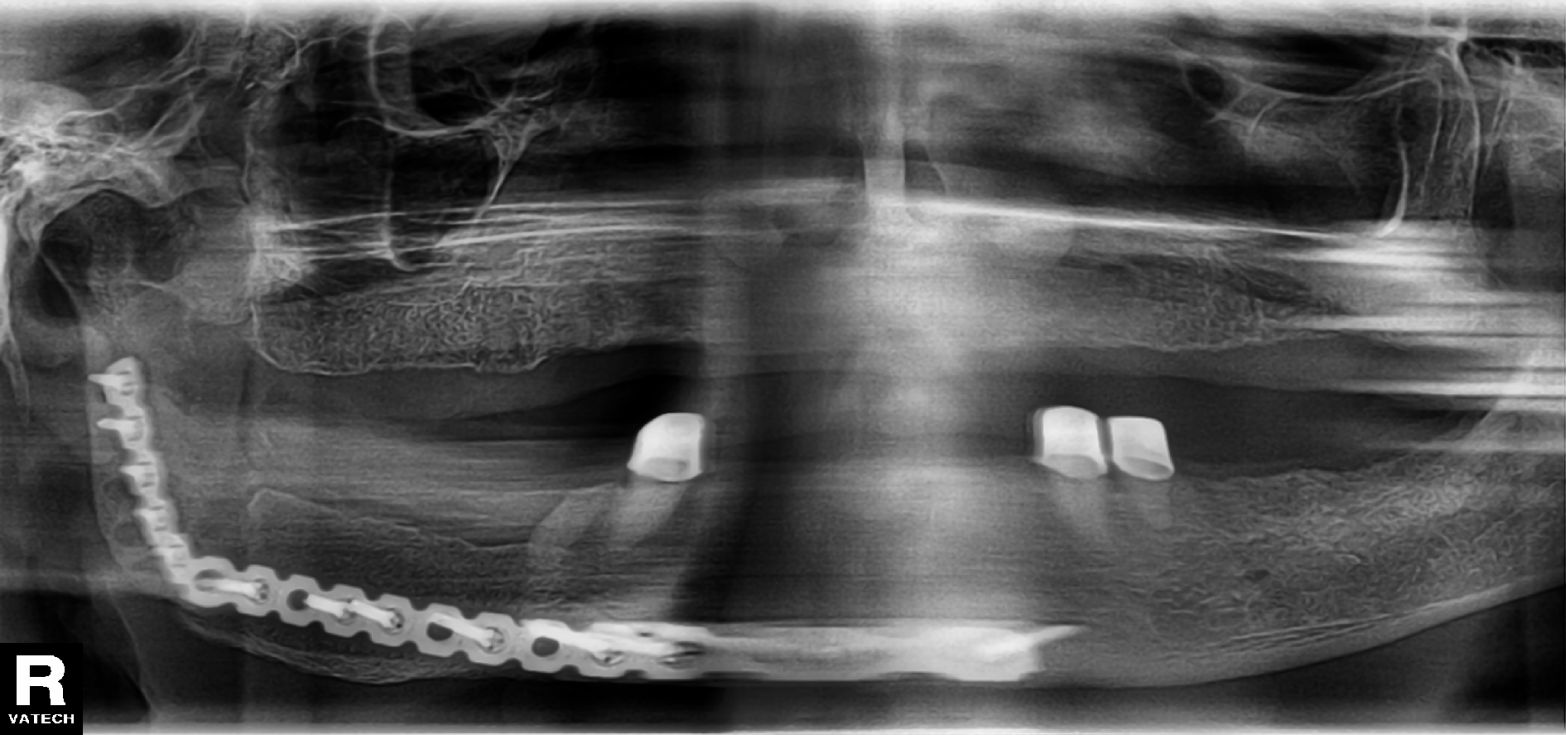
Panorex X-ray Case 1 - after secondary reconstruction of the mandible.
